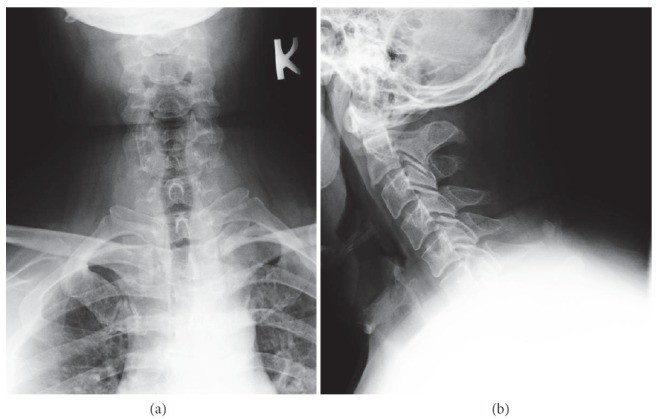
X-ray of the cervical spine. The foreign body is not visible in posteroanterior (a) and lateral (b) views.
